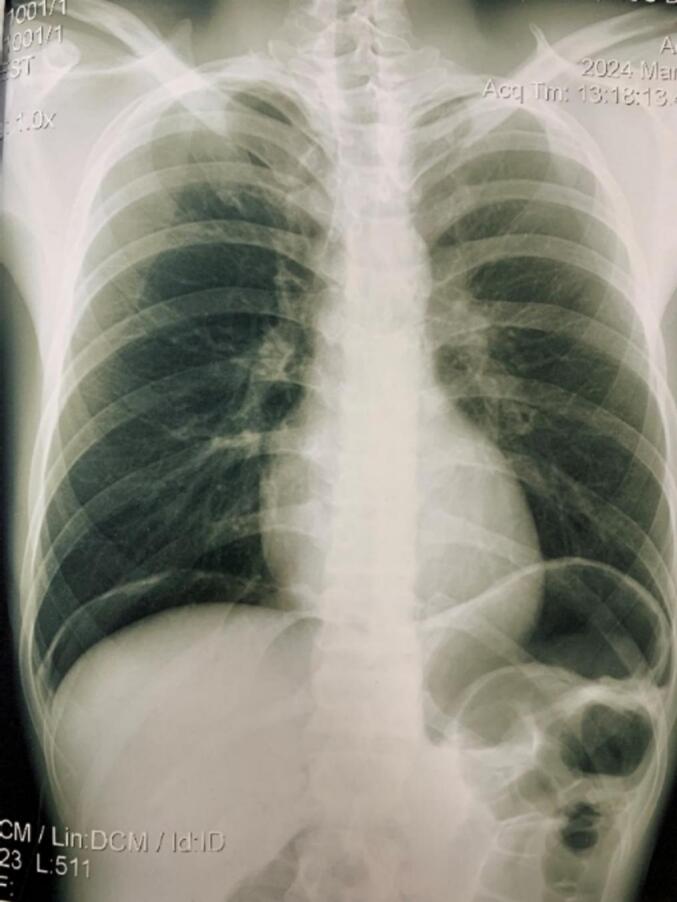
The chest X-ray after the colonoscopy showed a free air under the right diaphragm.
